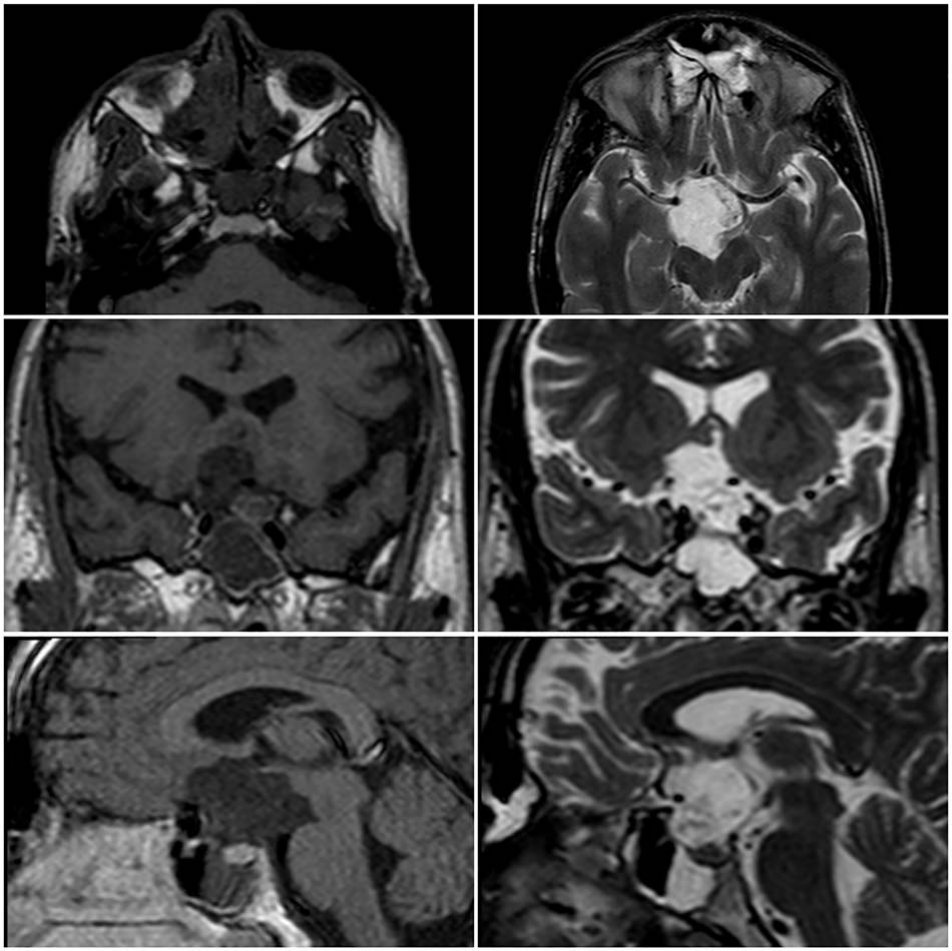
MRI in axial, coronal and sagittal views. The lesion appears with hyposignal in T1WI and hypersignal in T2WI.
